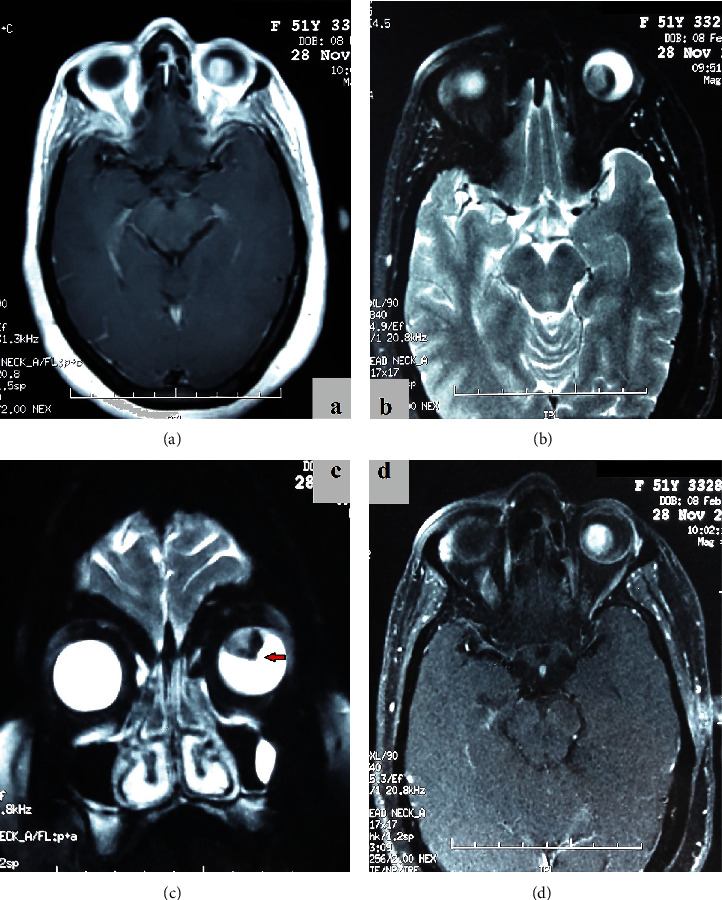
MRI of the orbits: (a) tumor showing high signal intensity on T1-weighted images; (b) heterogeneous appearance and low signal intensity on T2-weighted images; (c) T2-weighted images on a coronal plane: heterogeneous appearance and low intensity with a collar-button extension (arrow); (d) tumor shows enhancement on T1-weighted images with fat suppression, after Gadolinium administration.
